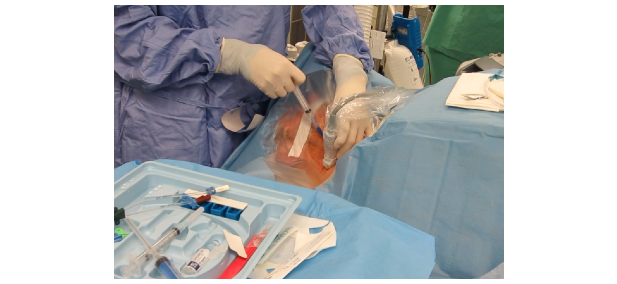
One-handed aspiration while holding the ultrasound probe.
